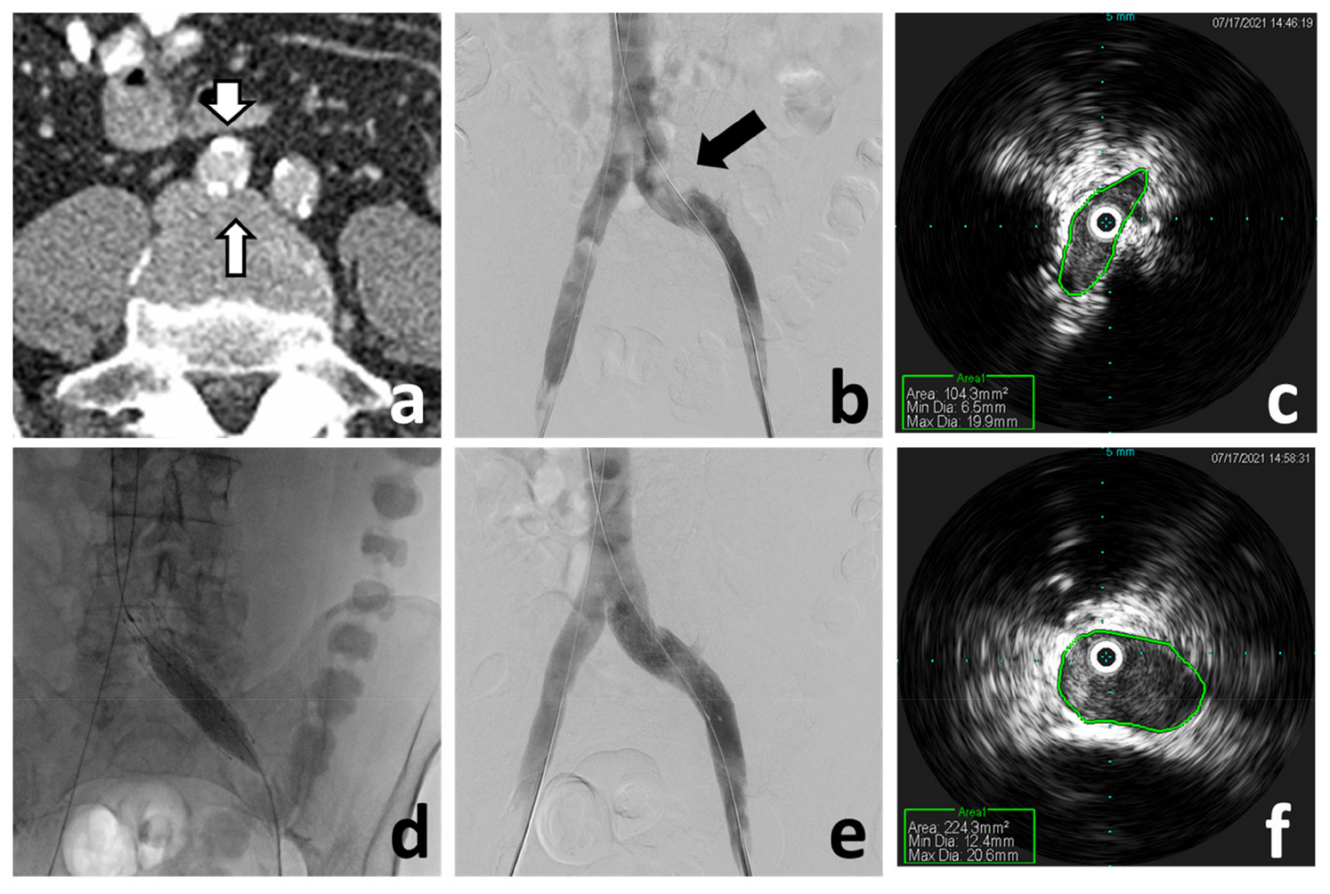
May–Thurner anomaly diagnosis and endovascular treatment. (a) Abdomen-pelvis computed tomography angiography demonstrates compression of the left common iliac vein (narrow arrow) by a calcific right common iliac artery (wide arrow). (b) Venography demonstrates the external compression of the left common iliac vein (arrow), although it does not accurately represent the severity. (c) Intravascular ultrasound (IVUS) of the left common iliac vein demonstrates the significant lumen reduction (area = 104.3 mm^2^). (d) Balloon post-dilatation of the deployed left iliac vein stent. Venography (e) and IVUS (f) post-stenting demonstrate correction of the external compression and stenosis (area = 224.3 mm^2^).
